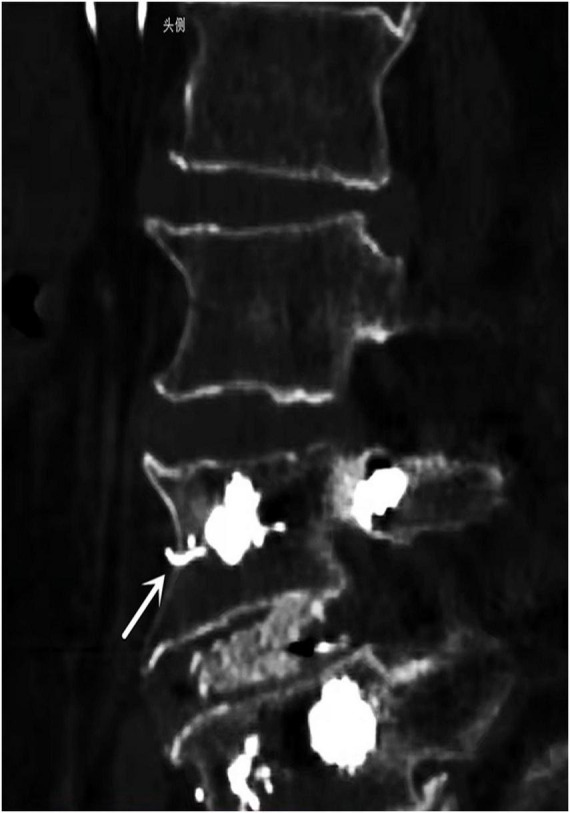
CT scans revealed strip-like high density cement extravasation (arrows) in vertebra bone.
